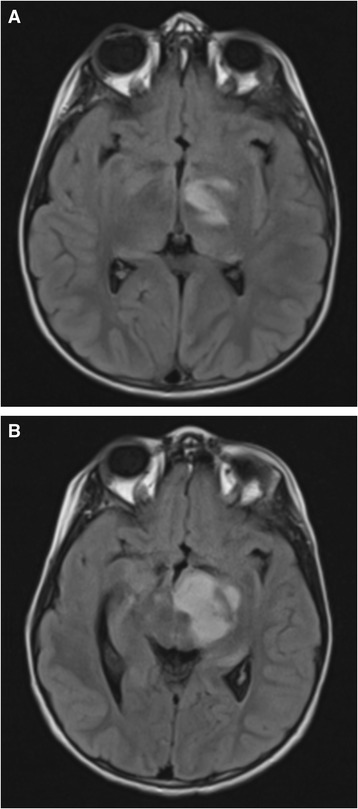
Progressing glioma in Patient 9. a First scan with glioma present in the left cerebral peduncle and left thalamus on FLAIR sequence. b Another scan performed 2 years after the previous one (again FLAIR sequence). The glioma has drastically increased in size and was treated with chemotherapy 1 month after this image was taken
